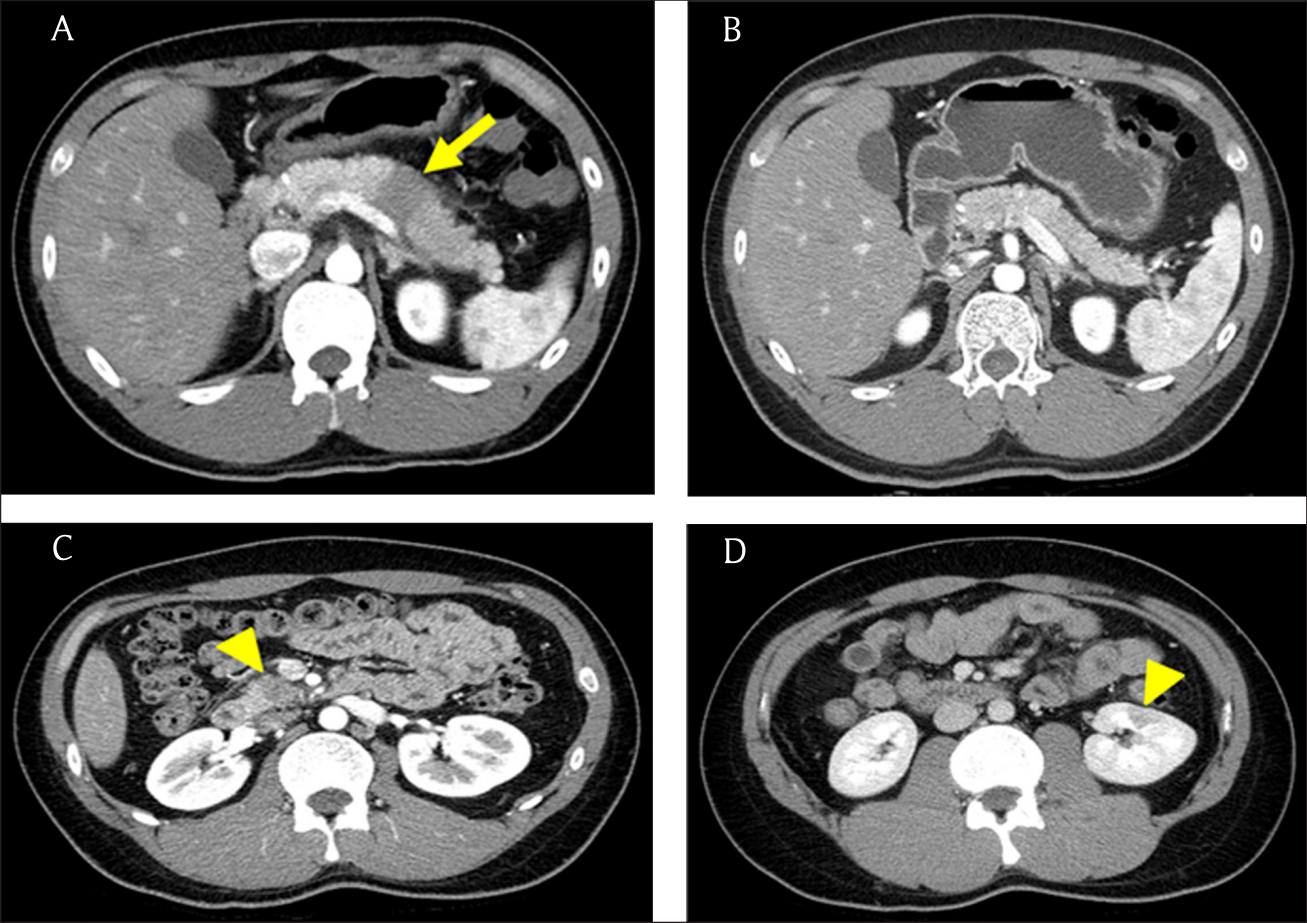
A 23-year-old man with focal type autoimmune pancreatitis. (A) Axial pancreatic phase CT image shows a low attenuating lesion (arrow) in the pancreatic body. Note no pancreatic duct dilatation in the tail portion. (B) Axial pancreatic phase CT image obtained after 3 months shows the disappearance of the mass. (C, D) On follow-up CT image obtained after seven months, newly developed low attenuating lesions (arrowheads) are noted in the uncinate process of the pancreas and the left kidney.
